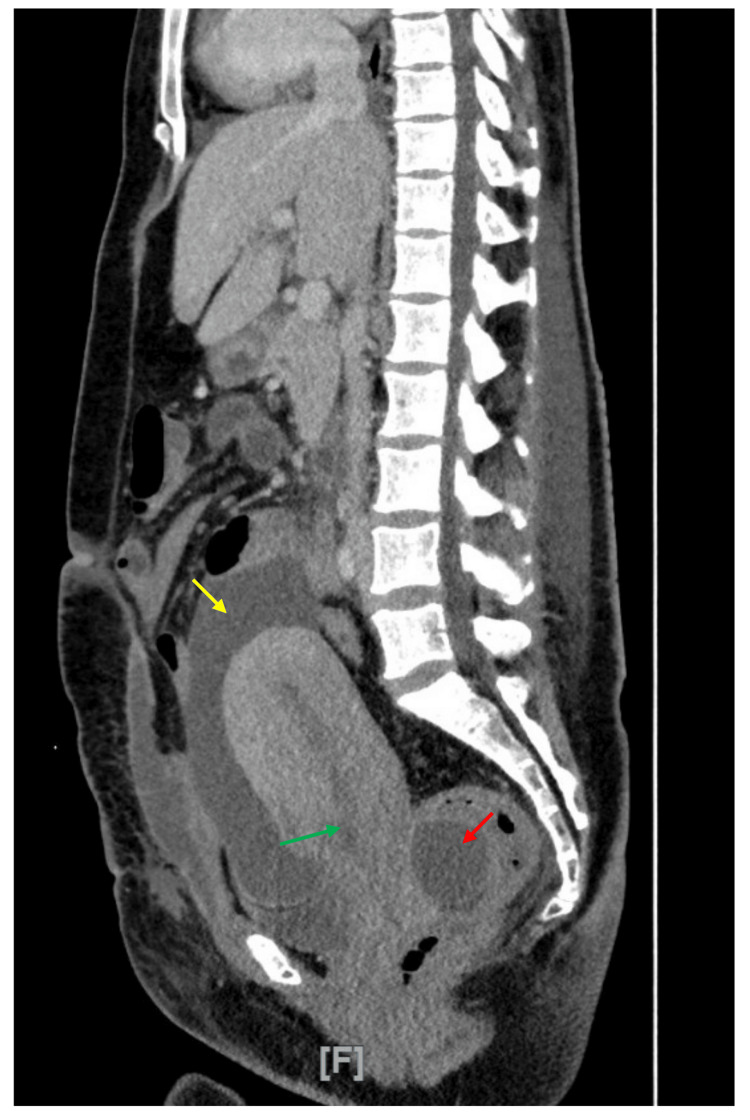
Repeat sagittal contrast-enhanced CT of the abdomen and pelvis on day 6 Yellow arrow indicates localized collection posterior to the cervix with a thick enhancing wall measuring approximately 6.6 x 3.8 cm. Green arrow indicates hypoattenuation defect in the anteroinferior uterine wall consistent with a cesarean section scar. Red arrow indicates larger fluid collection superior and anterior to the uterus.
